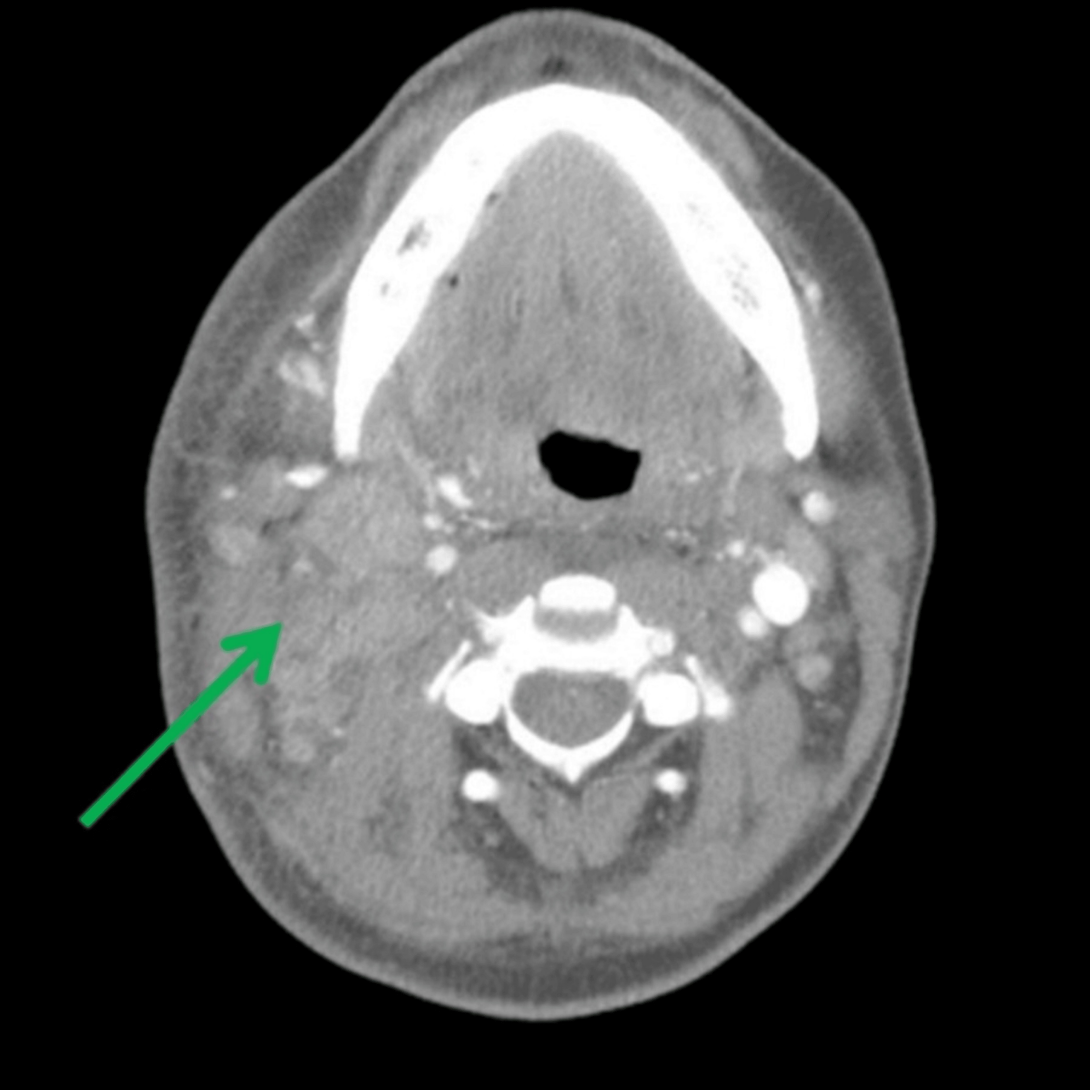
CT neck with contrast demonstrating increased diffuse metastatic cervical lymphadenopathy right (green arrow) greater than left.
